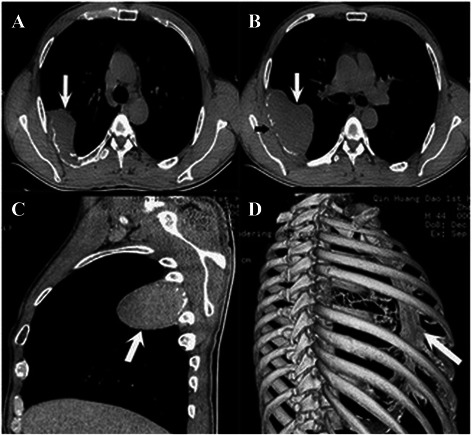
Spiral computed tomography showing more detailed imagery and data. Three-dimensional computed tomographic image of the chest showing an irregular mass projecting into the thorax (*arrow*, a, b and c) and a focal lytic lesion in the sixth rib (*black arrow*, b). Spiral CT 3D reconstruction showing the absence of the right sixth rib (*arrow*, d)
